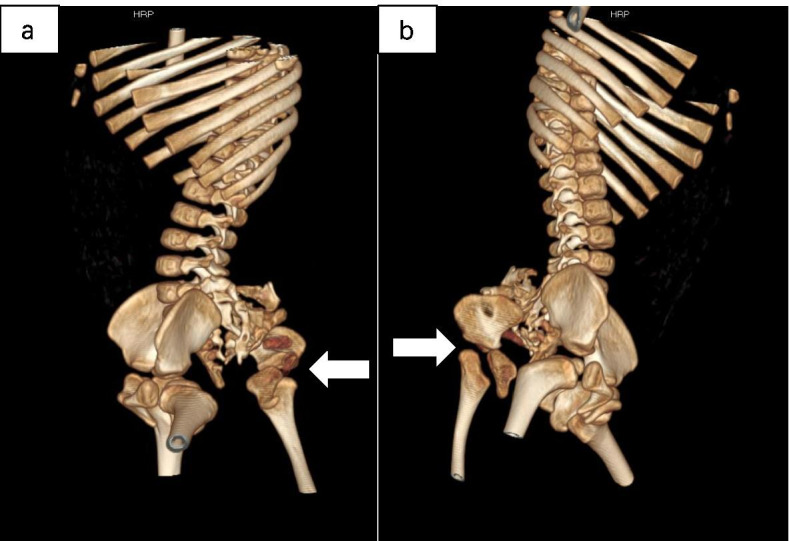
a and b 3D CT images showed the bony structure in the mass resembling sacrococcygeal bone, hip bone and the femur (thick arrow)
